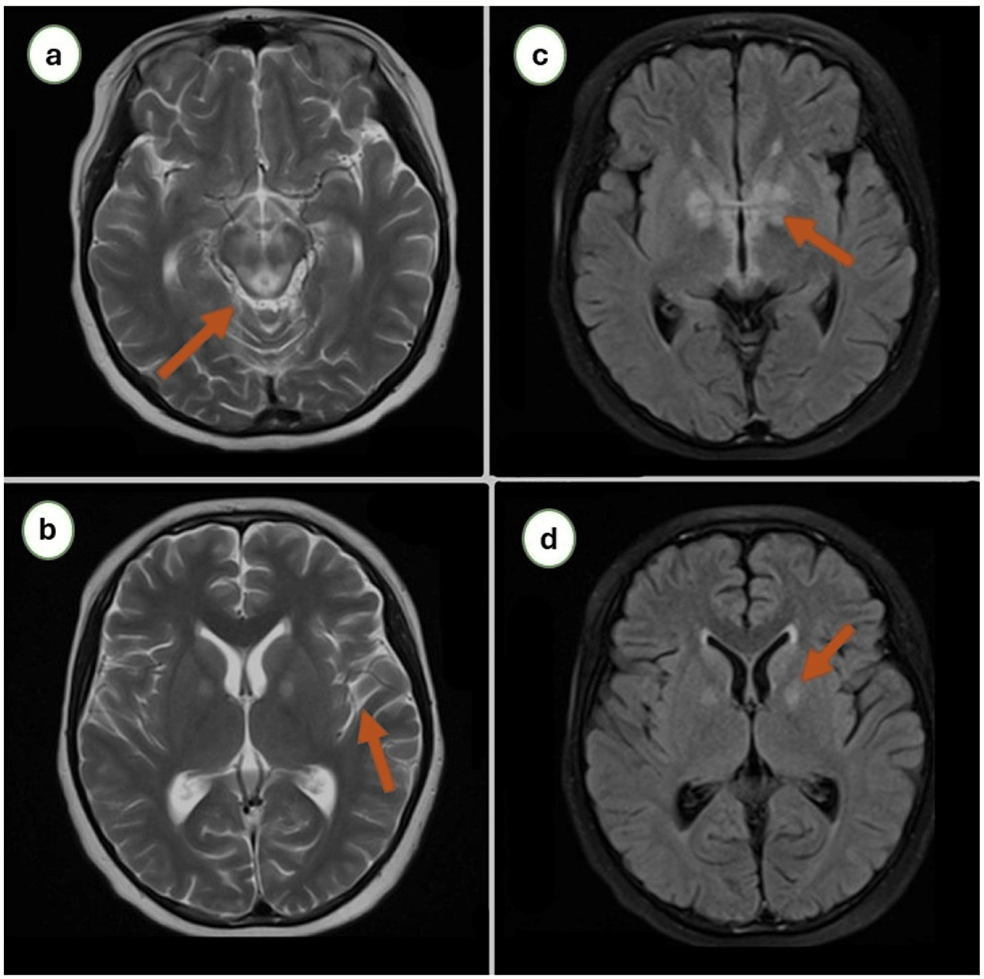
Brain MRI with T2-weighted (a,b) and diffusion-weighted (c,d) images showing marked bilateral signal abnormalities in the basal ganglia, brain stem, and brain white matter.
